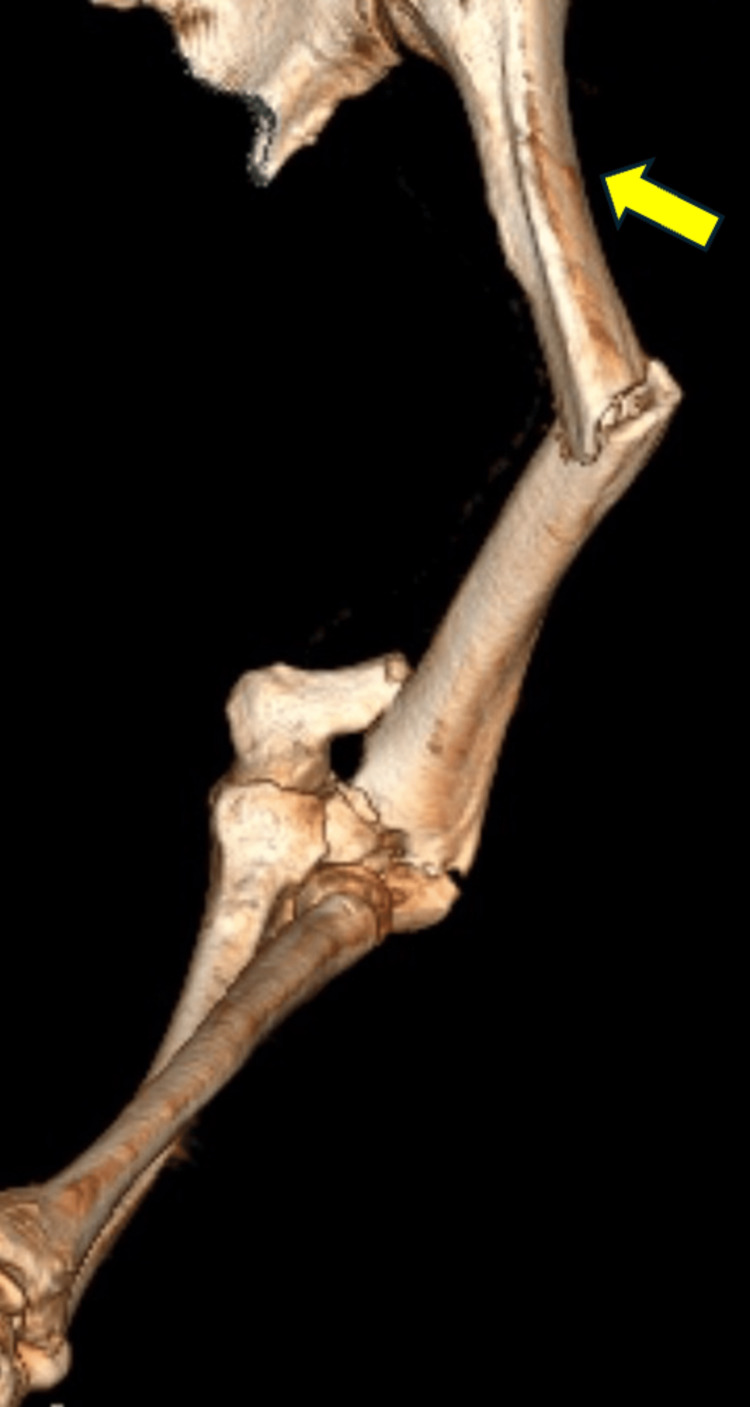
3D reconstruction of the CT scan depicting the radial head fracture and the supra-intercondylar, midshaft, and proximal incomplete humeral fractures. The incomplete fracture is shown with a yellow arrow.
